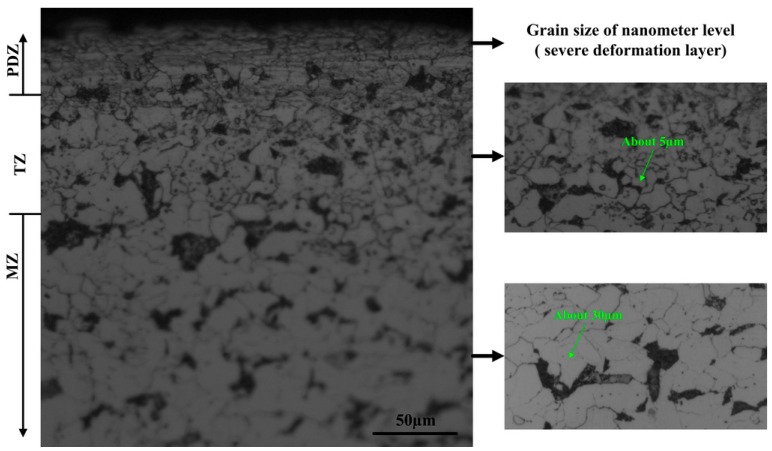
Microstructure and deformation layer after Ultrasonic peening treatment (UPT) (PDZ: Plastic deformation zone; TZ: Transition zone; MZ: Matrix zone).
